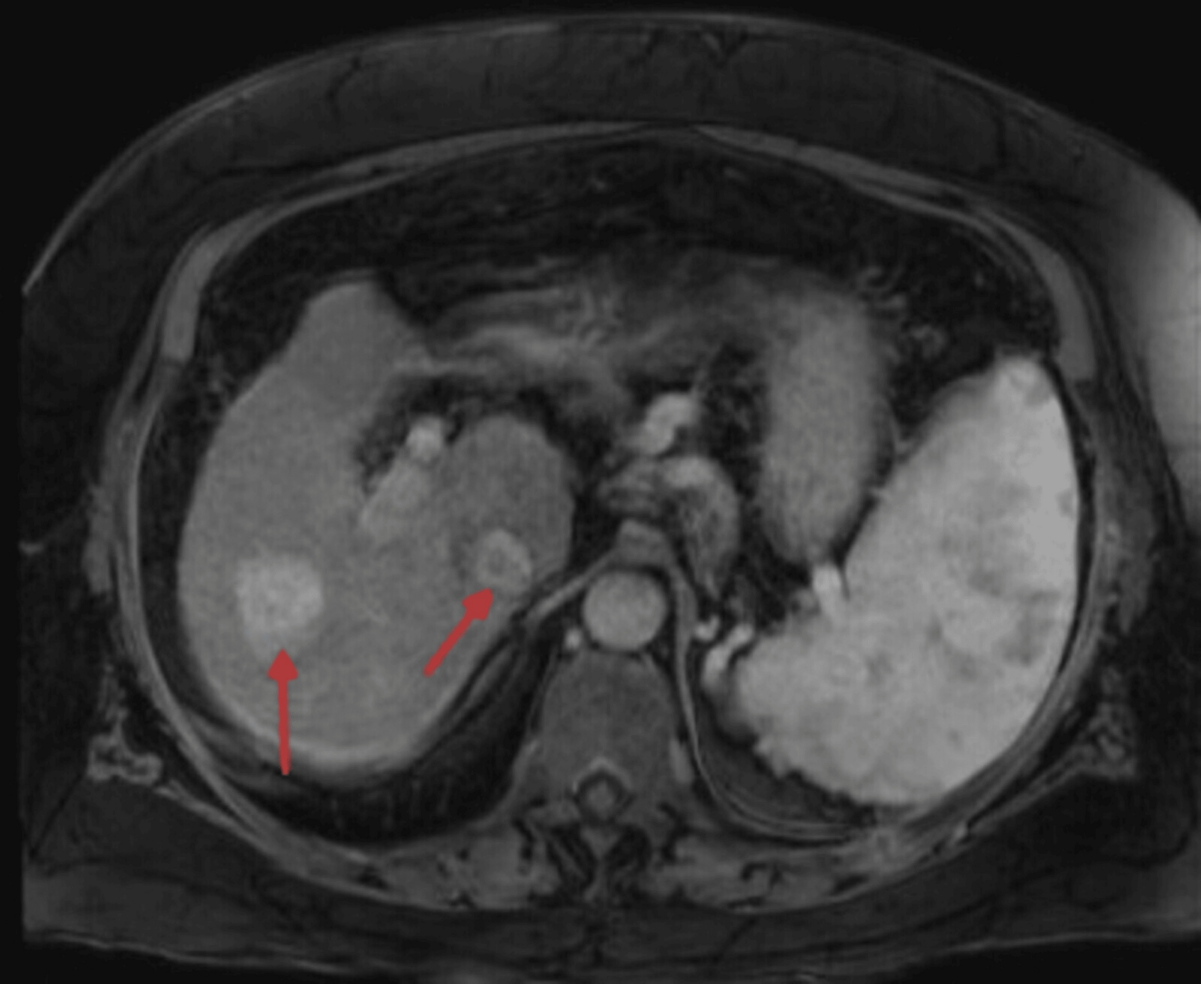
Preprocedural magnetic resonance imaging (MRI) of the liver demonstrating hepatocellular carcinoma. Axial contrast-enhanced T1-weighted imaging shows a 3.4-cm arterially enhancing lesion at the junction of hepatic segments 7 and 8, with intrinsic T1 hyperintensity and imaging features consistent with a Liver Imaging Reporting and Data System (LI-RADS) 5 hepatocellular carcinoma (red arrows). Interval growth on serial imaging prompted consideration of locoregional therapy as a bridge to liver transplantation.
